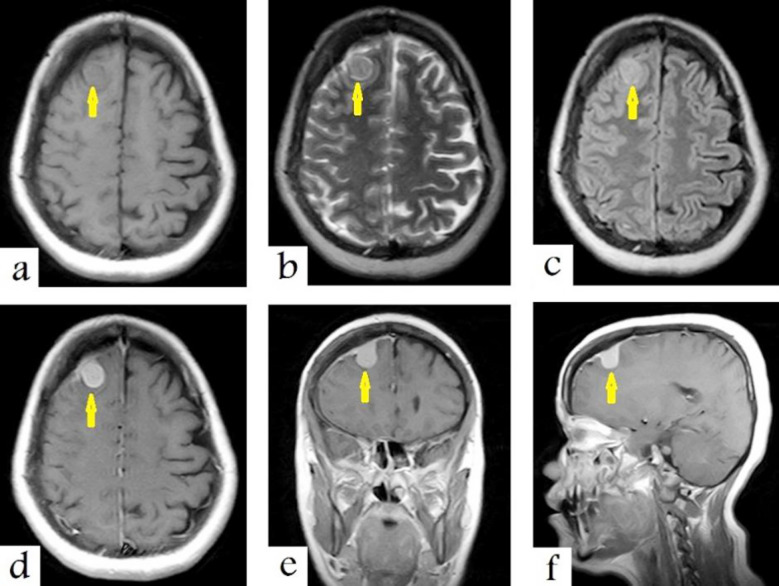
Selected images of the brain MRI show a well-defined extra-axial tumuor of approximately 26×17 mm in the right frontal lobe. It appears isointense on T1-weighted images (a), sight hyperintense on T2-weighted images (b) and fluid-attenuation inversion recovery (FLAIR), (c) with avid enhancement and a dural-base and tail on T1-weighted images after contrast administration, (d, e and f). This strongly suggests right-side convexity meningioma (arrows).
